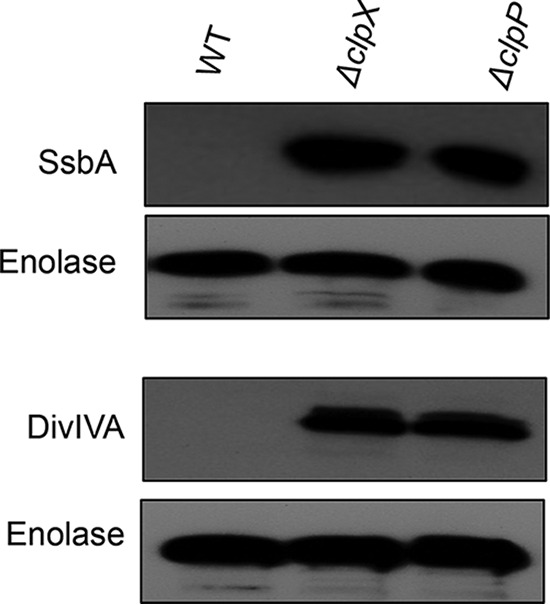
SsbA and DivIVA proteins are degraded by ClpXP in *S. mutans*. *ssbA* and the DivIVA gene were cloned in pIB190 vector in N-terminal histidine-tagged form and were transformed in the wild-type (WT) UA159 strain and its isogenic Δ*clpX* and Δ*clpP* mutant strains. After cell lysis, equal amounts of protein (~20 µg) were loaded in all lanes. The amounts of SsbA and DivIVA present in each strain were evaluated using anti-His antibody. The blots were developed with a chemiluminescent kit (ECL Plus; Thermo Scientific) by exposure to X-ray film and then photographed. Endogenous enolase was used as a loading control. Experiments were repeated at least three times, and the respective areas of a representative blot are shown.
